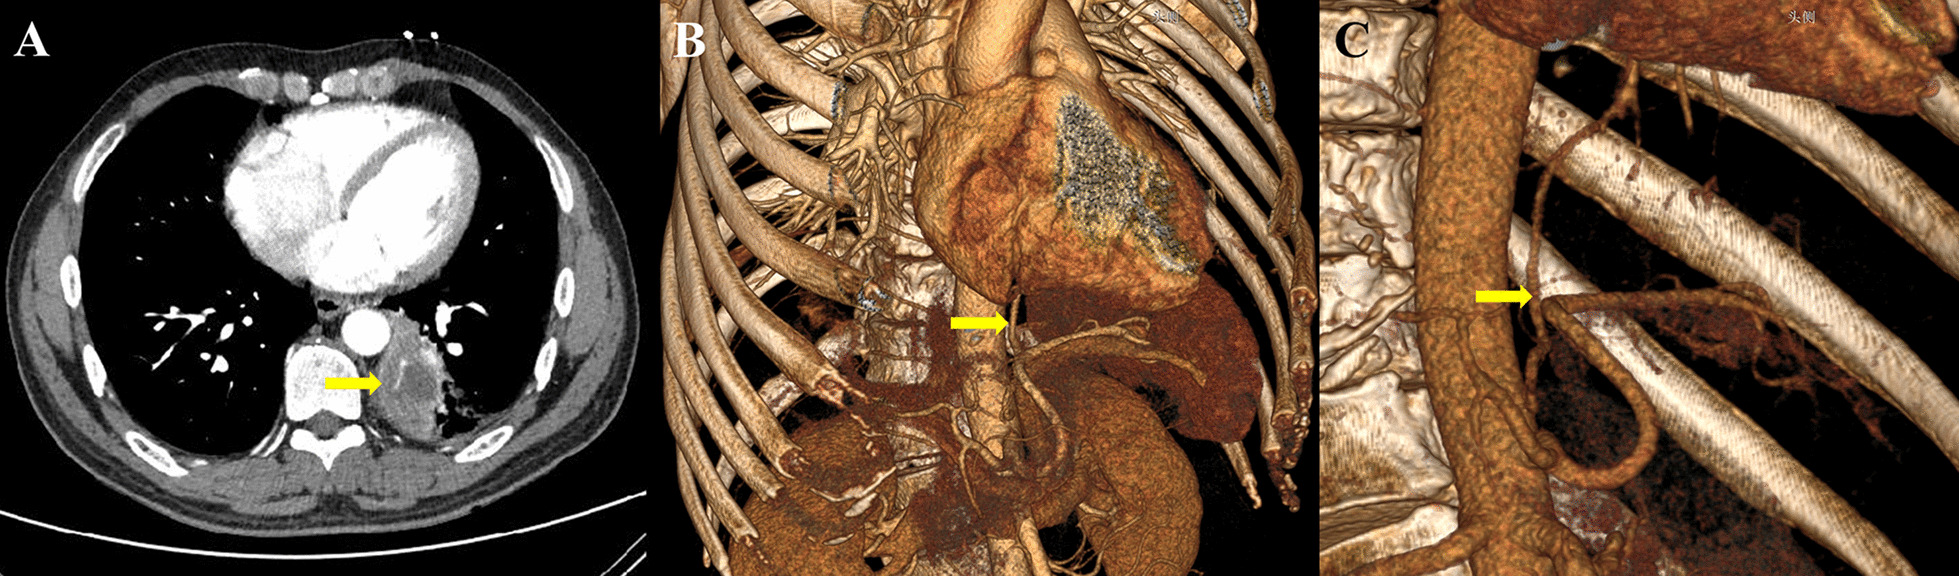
Three-dimensional computed tomography angiography showed definitely the anomalous blood supply. A A mass was located in the left lower lobe; B the abnormal feeding artery was next to the spleen artery; C the feeding artery originated from the abdominal aorta was finally confirmed
